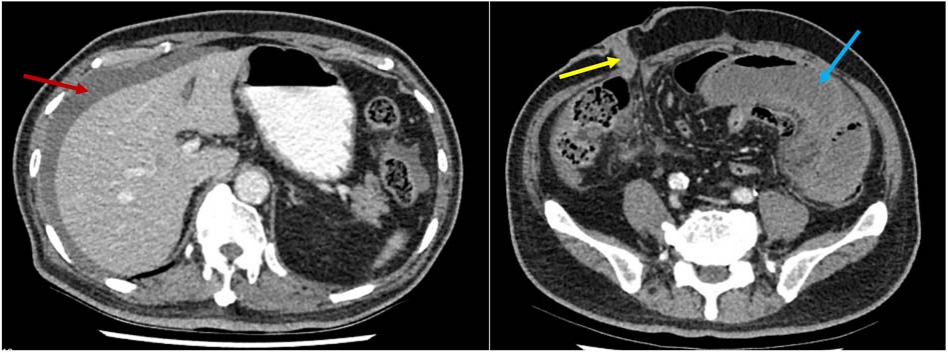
CT scan axial slices demonstrating perihepatic free fluid (red arrow) and dilated loops of small bowel in the left iliac fossa (blue arrow). The ileal conduit (yellow arrow) in the right iliac fossa appears healthy.
